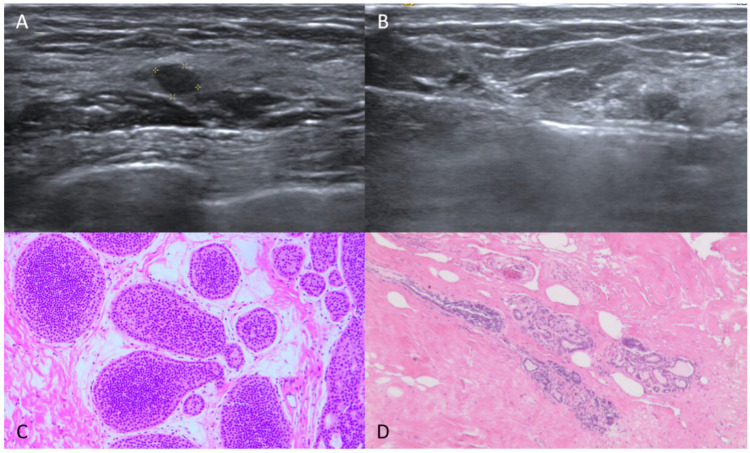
A 45-year-old lady with a hypo-echoic lesion in the left breast. (A) The tiny lesion (5 × 4 mm, asterisks mark the extent of the lesion) is oval, with a circumscribed margin. It was categorized as BI-RADS 3. (B) Ultrasound-guided vacuum-assisted excision was performed. (C) Pathology showed low grade ductal carcinoma in situ. (D) Post-surgery showed no residual carcinoma.
